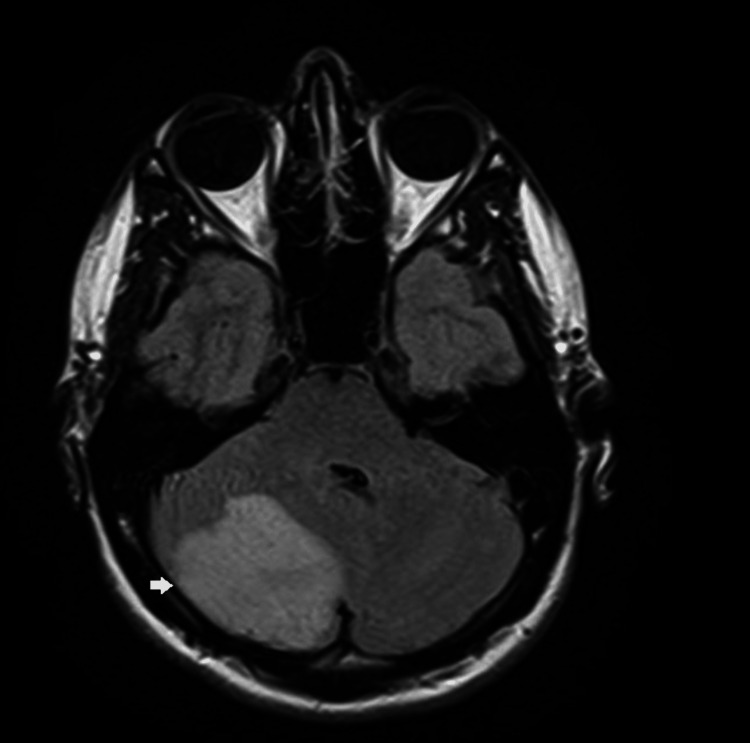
MRI brain (axial view) showing large right cerebellar ischemic stroke (white arrow)
